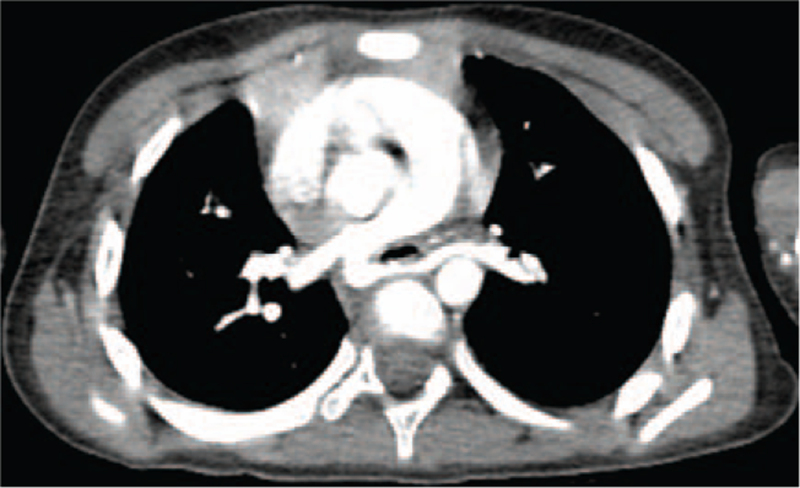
CT angiography depicting the origin of the left pulmonary artery arising from the posterior aspect of the right pulmonary artery and travelling between the esophagus and trachea towards supplying the left lung.
